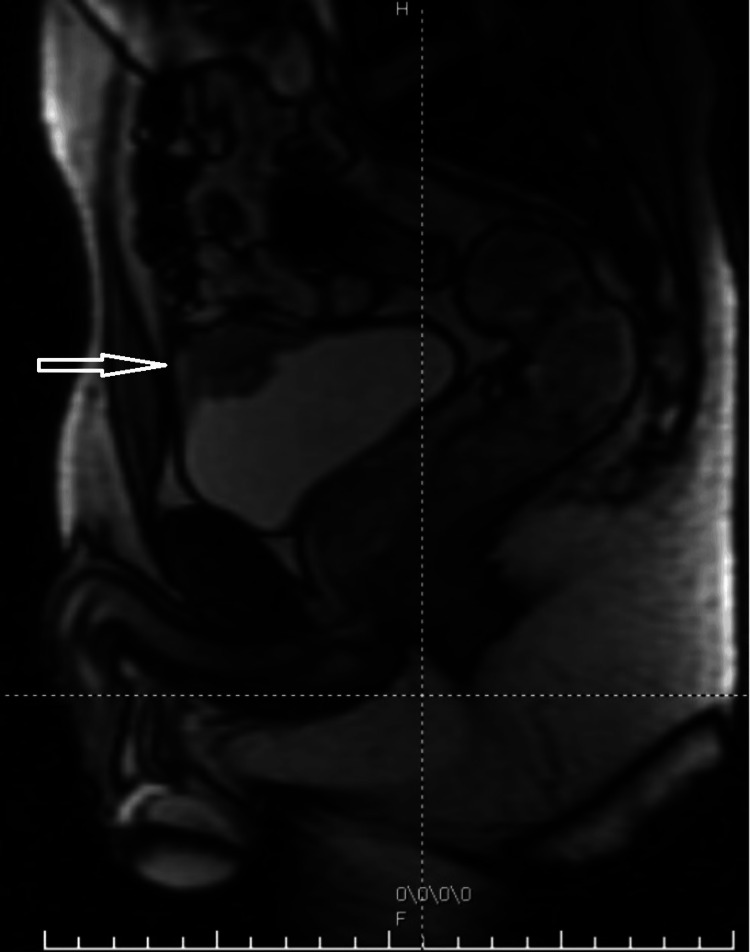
MRI pelvis (sagittal view) The image demonstrates an apical bladder tumour involving a urachal remnant.
